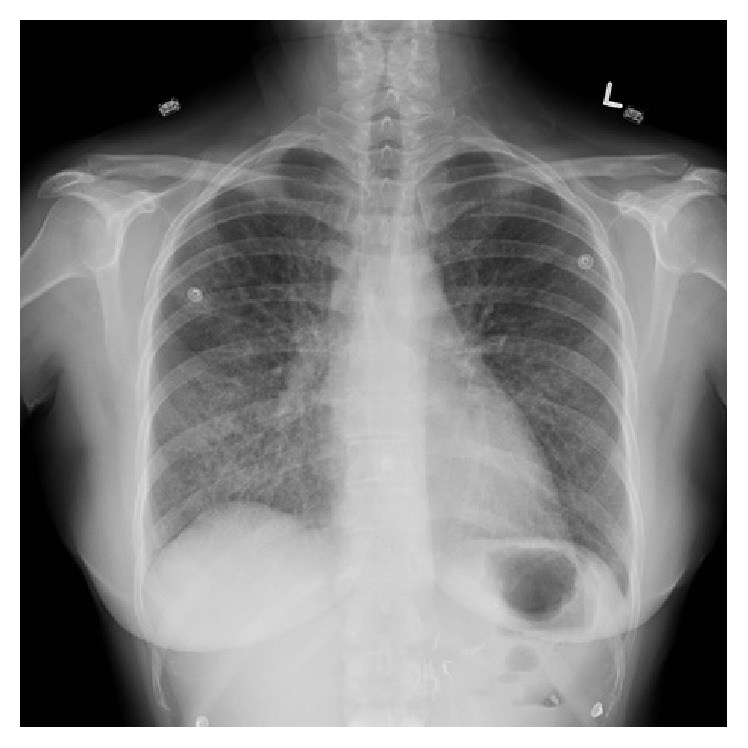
Posterior-anterior chest radiograph after three sessions of WLL, demonstrating improvement of the infiltrative process.
